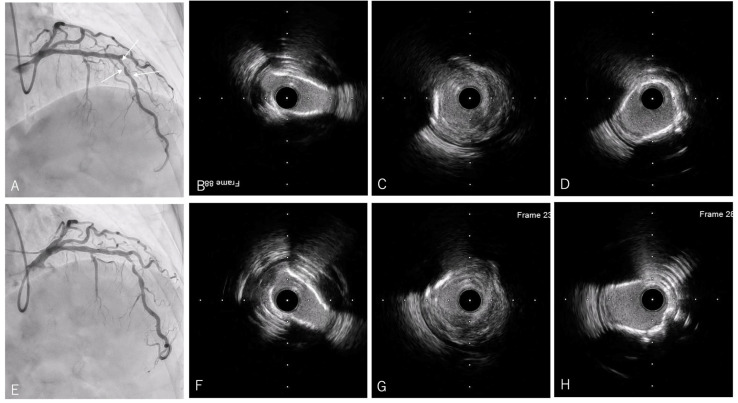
OA with IVUS guidance in a severely calcified LAD lesion. (A) Angiogram. (B–D) Intravascular ultrasound (IVUS) demonstrating severe calcification in the proximal and distal segments. However, the guidewire position was not good for OA (10 o’clock) in the midportion. The IVUS marking method identified the OA sites in the proximal and distal segments and no touch in the midportion. (E–H) Angiogram after OA at 80,000 rpm. Lumen gain could be obtainedand reverberations occurred from 12 to 2 o’clock and 6 to 8 o’clock in the proximal lesion. Lumen gain could be obtained, and reverberations occurred from 12 to 2 o’clock in the distal lesion. In the midportion, OA crown was passed with Glideassist mode. No significant change was observed in the midportion. White arrows indicate the calcified and non-calcified lesions.
